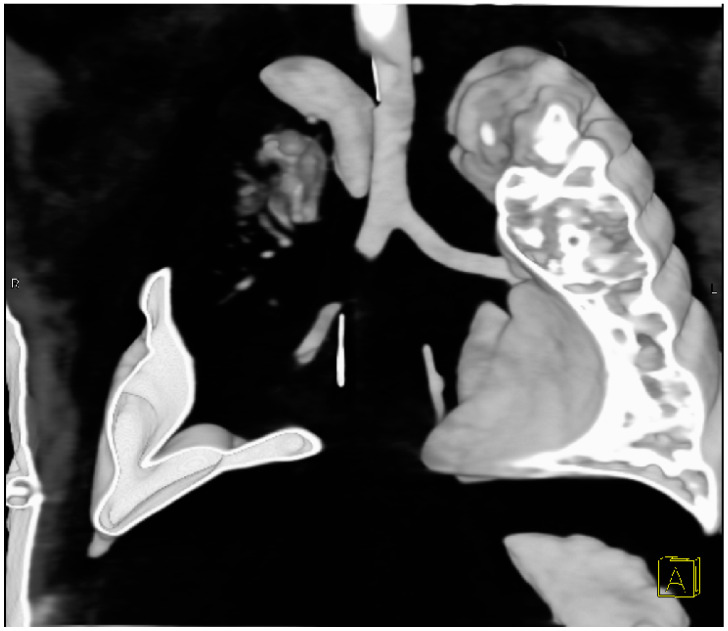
CT bronchography—occlusion of the right main bronchus 6 mm distal to the bifurcation, extending 8 mm.
